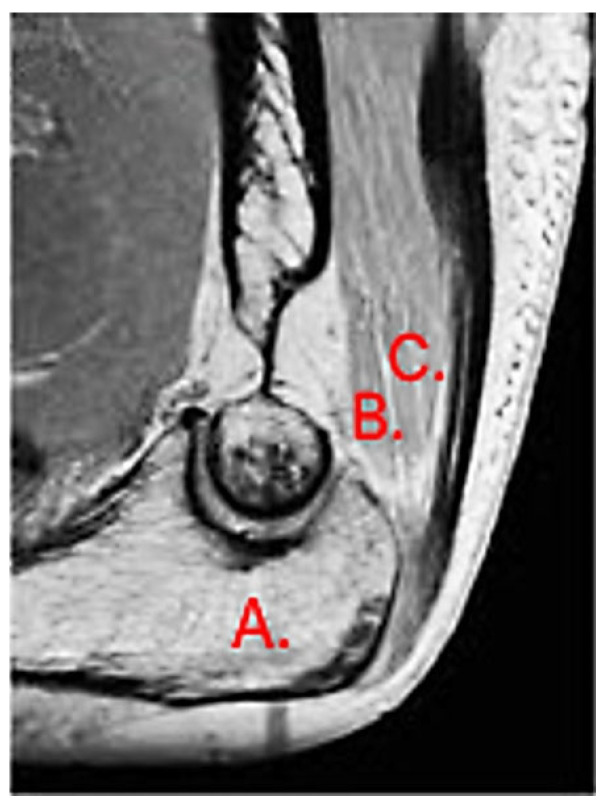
Normal anatomic attachment relationships of the triceps tendon in sagittal section to the olecranon ulnae. MRI: A—olecranon, B—medial head, C—common tendon of lateral and long head (own source).
